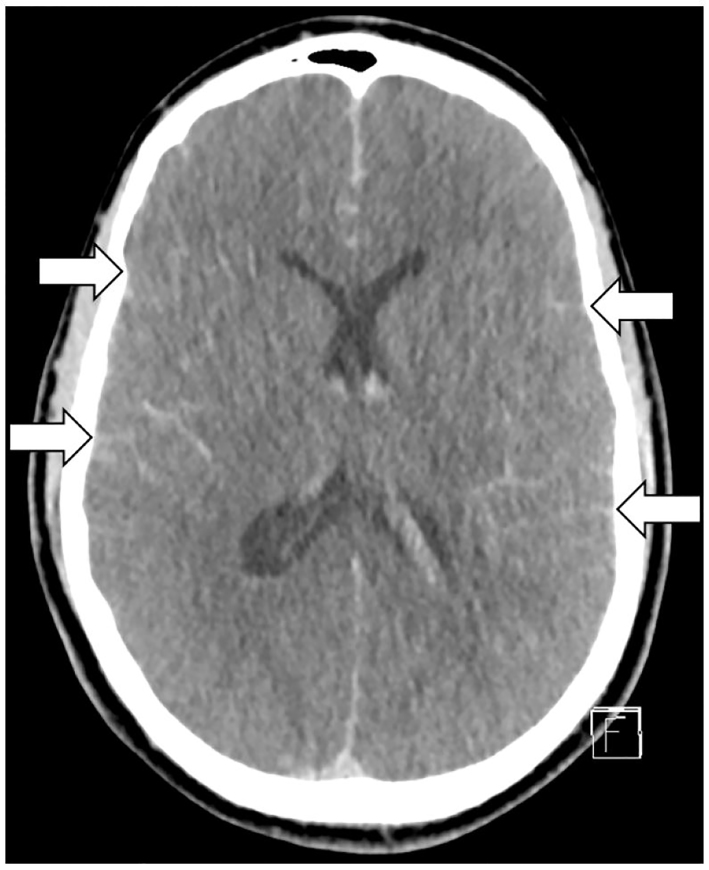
Cerebral computed tomography, showing diffuse subarachnoid hemorrhage with generalized cerebral edema.
